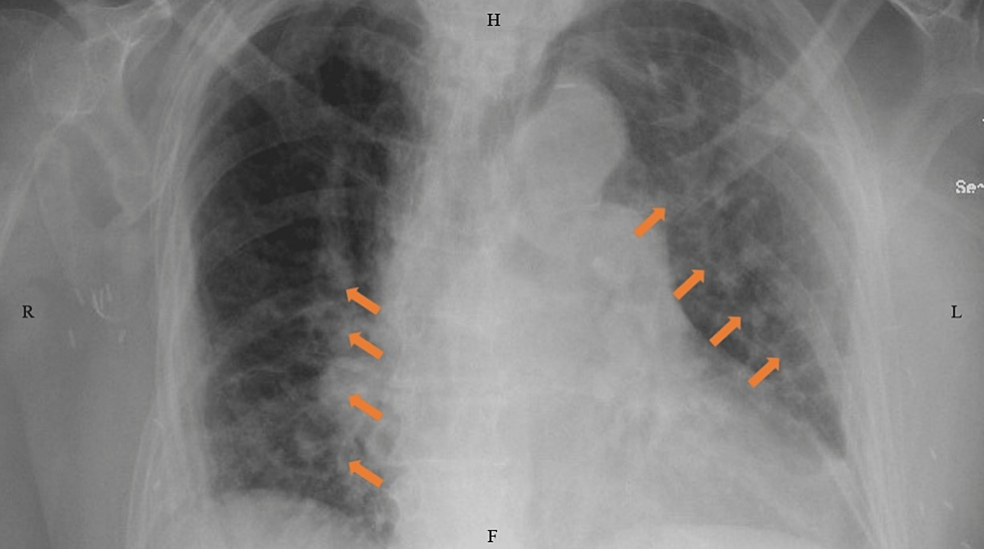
Chest x-ray showing interstitial edema (arrows). R: right; H: head end; L: left; F: foot end
